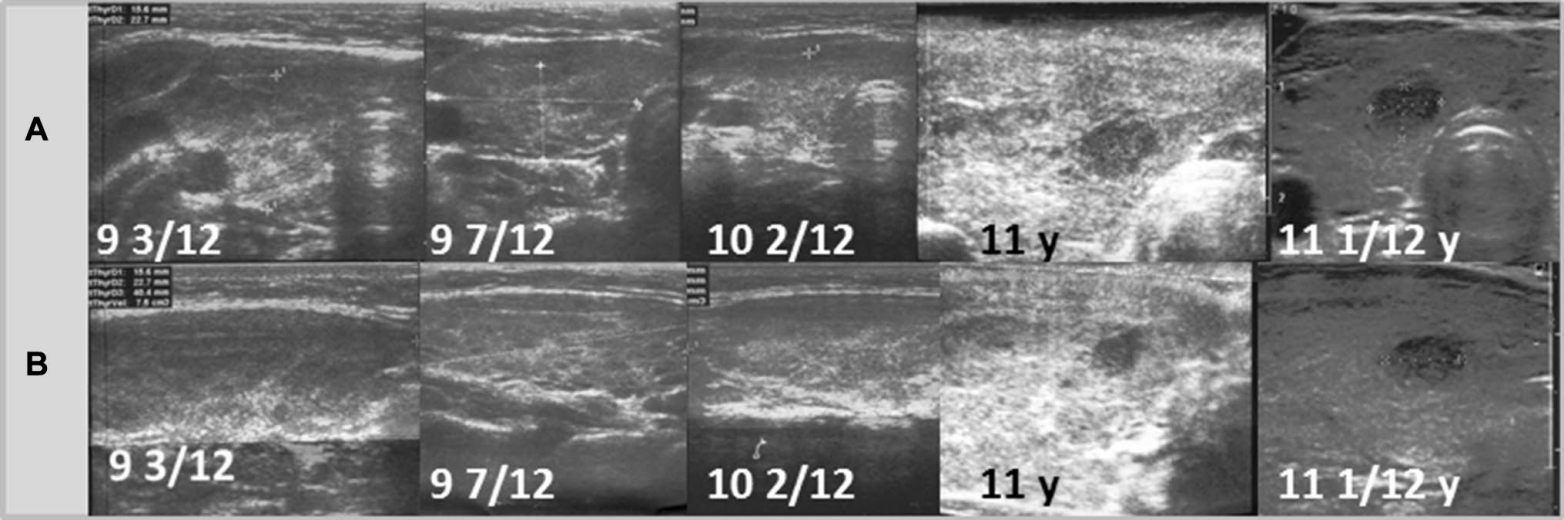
Patient 2. Transversal (a) and longitudinal (b) scans of the hypoechogenic thyroid gland revealed the formation of the nodule 1 9/12 years since referral and 10/12 months since last ultrasound assessment. The growth of the nodule was fast from 5.4 × 4.7 × 4.2 mm to 6.5 × 4.7 × 6.7 mm in 1 month (92% increase). During follow-up, the echogenicity of the thyroid gland increased
